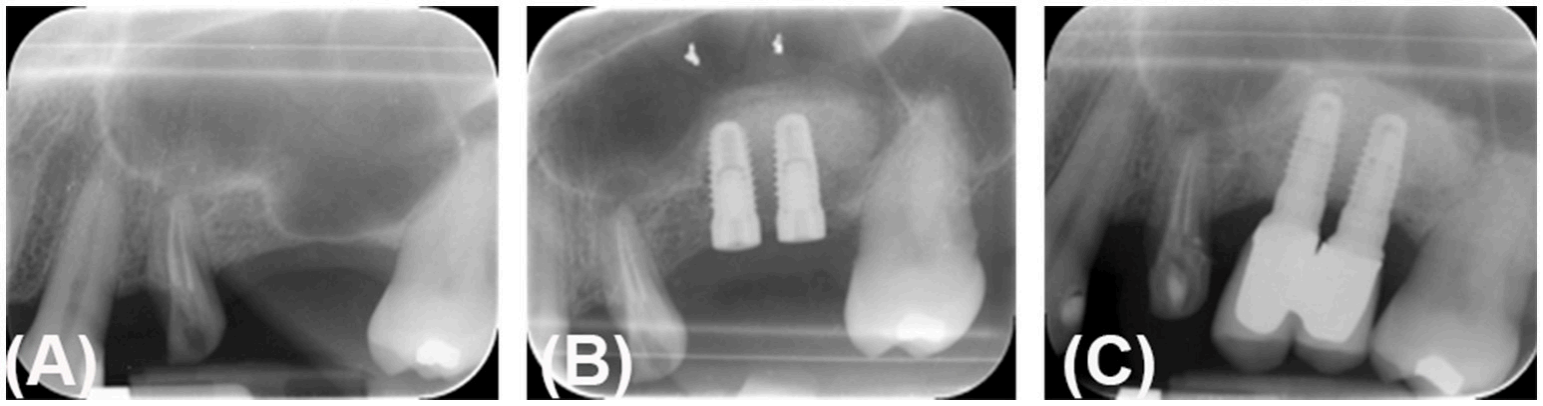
Radiography control after 6 months of micrograft application in patients treated with PLGA/HA + periosteum-derived micrografts. (A) Pre-surgical Rx. (B) Implant surgery Rx after 4 months and Rx after 2 years (C).
